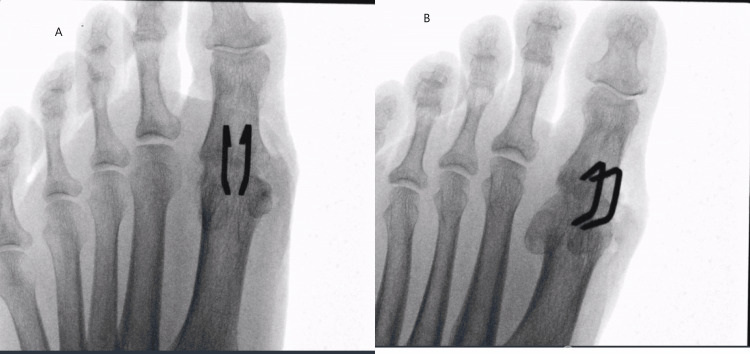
An intraoperative mini C-arm fluoroscope confirming the position of staples A: AP radiograph. B: Oblique radiograph AP: Anteroposterior
